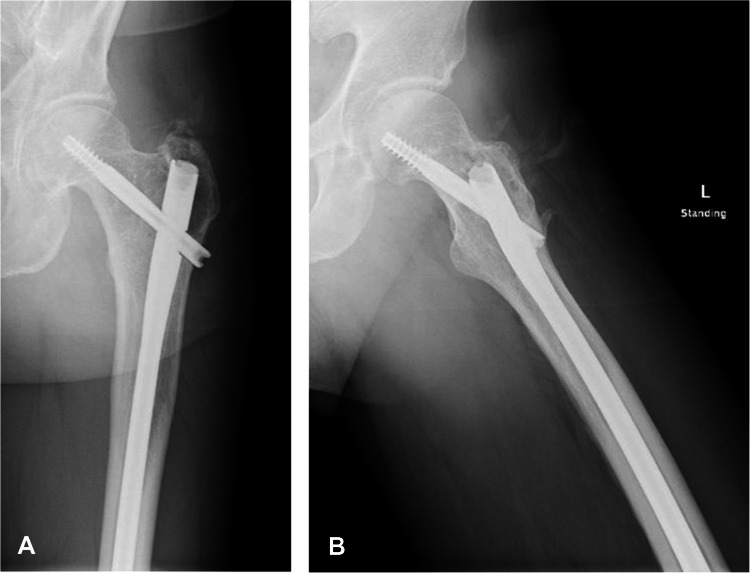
A, B Anteroposterior and lateral radiographs of the proximal and distal femur show significant improvement in the proximal diaphyseal femoral lesion, with reduced radiolucency and evidence of healing
